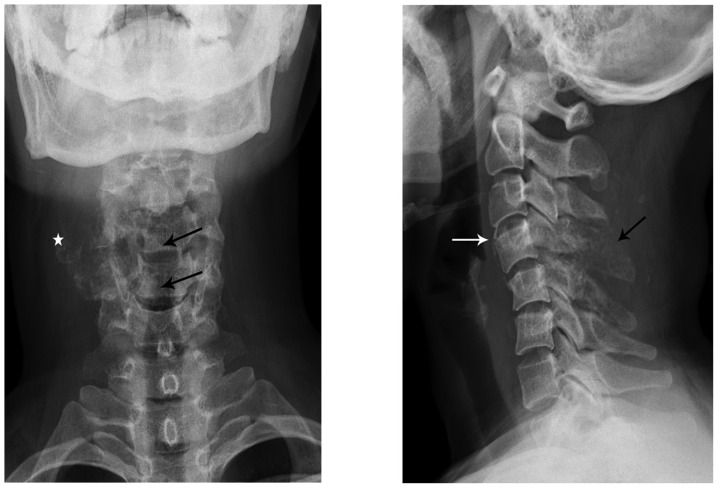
Antero-posterior and lateral view of the radiograph revealing the destruction of the spinous process of C4 and C5 (black arrow), and a soft tissue mass with calcification (pentagram). The front of the vertebral body had been infiltrated by the tumor (white arrow).
